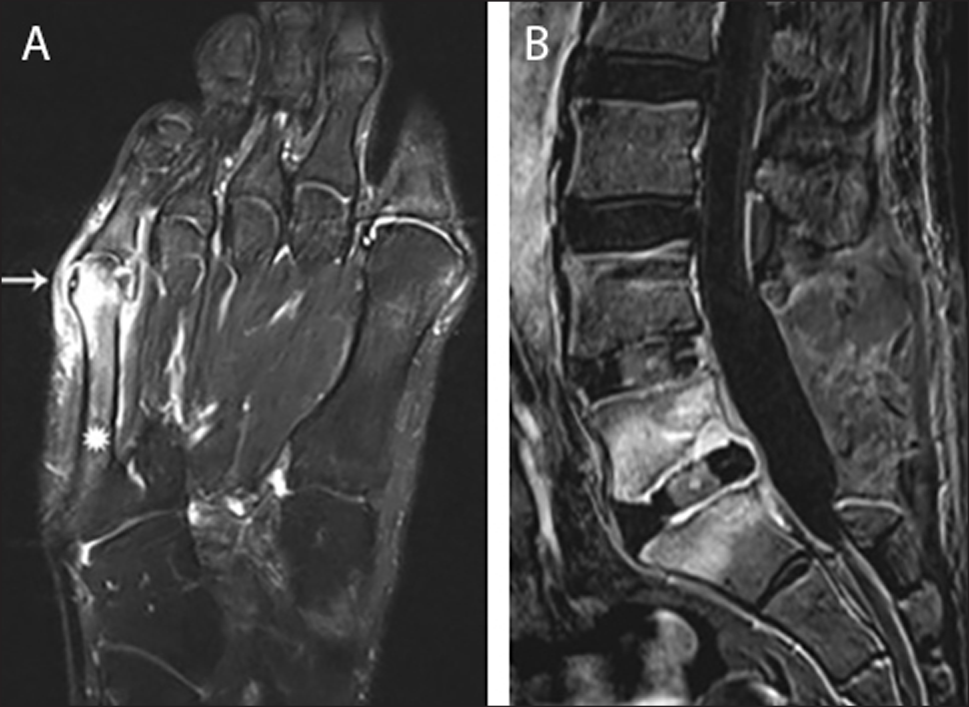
Main routes of infectious spread. (a) Infectious spread from contiguous infected tailors bunion. Axial T1-weighted image (WI) with Fat Suppression (Fat–Sat) after gadolinium contrast administration shows an inflamed bunion (white arrow) with adjacent enhancement of the distal aspect of the 5^th^ metatarsal (asterisks). (b) Postoperative infectious spread. Postoperative spinal infection. Sagittal T1–WI with Fat–Sat after gadolinium contrast administration. Note laminectomy L4 and L5 with placement of intervertebral cages. Extensive enhancement of L5 and S1 with rim enhancement of the intervertebral cage L5–S1 in keeping with postoperative spondylodiscitis.
